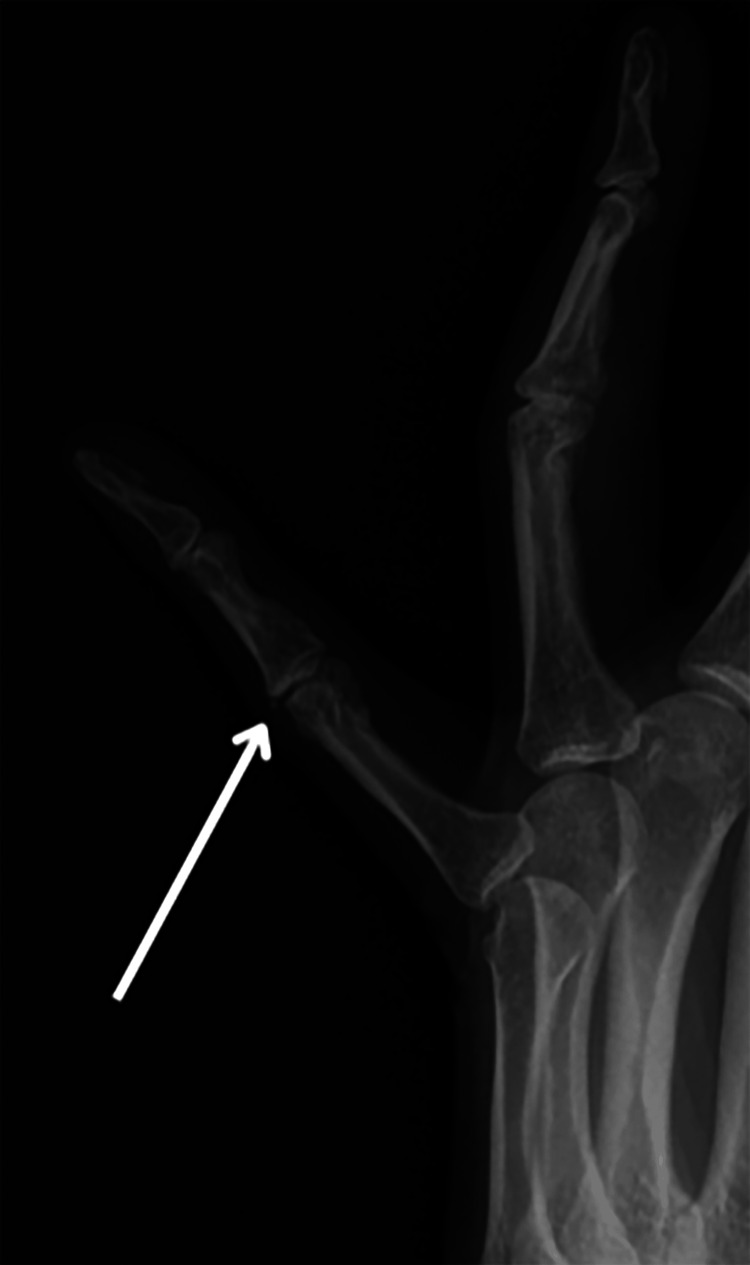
Bone avulsion previously seen on the dorsal aspect of the proximal interphalangeal (PIP) joint one year ago on X-ray (white arrow).
